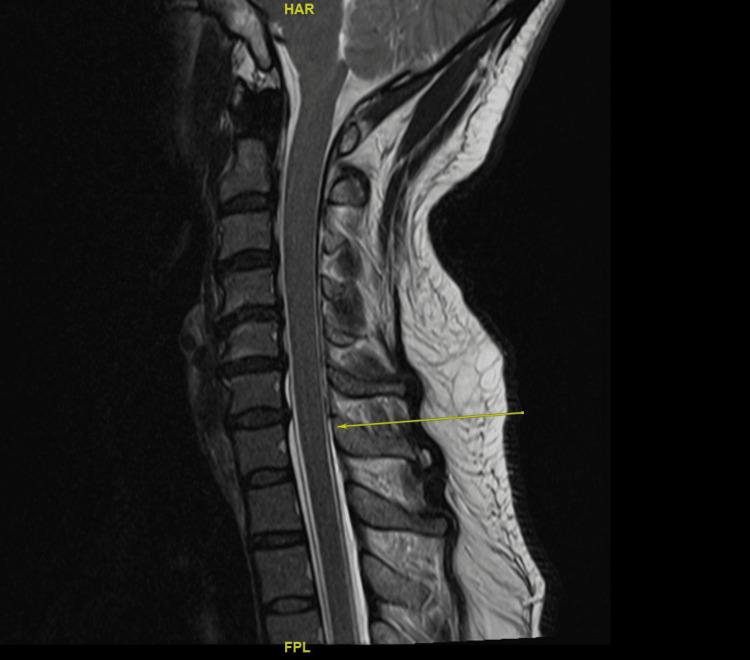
T2-weighted image showing epidural fluid collection extending from C6 down (yellow arrow).
